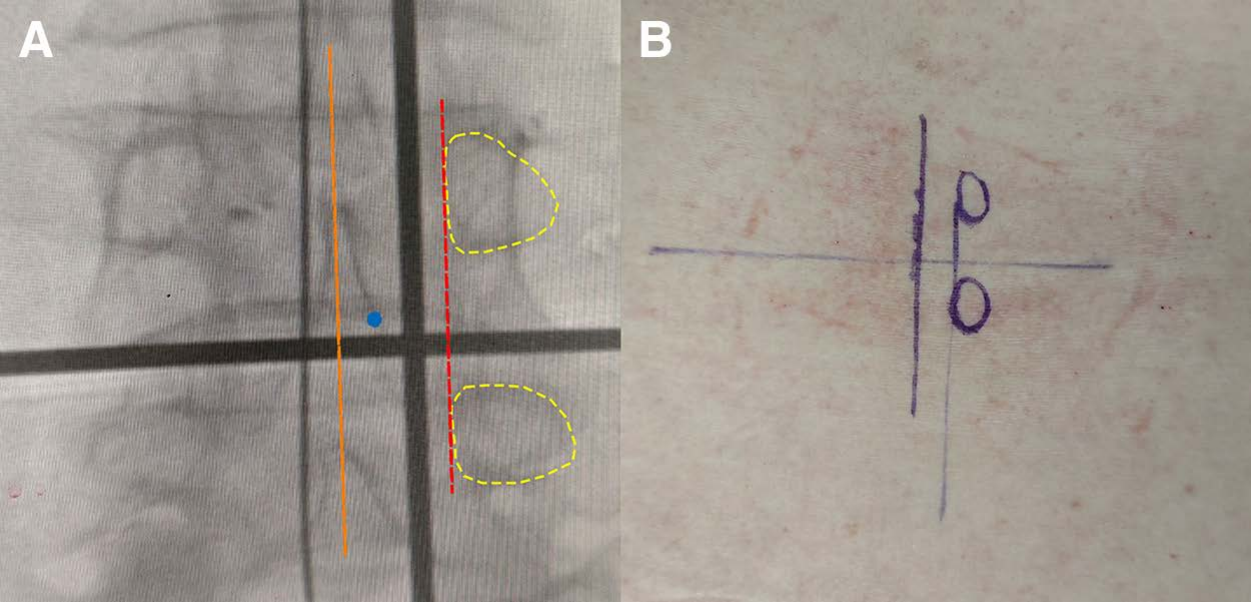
Preoperative X-ray localization and body surface marking. (A) Anteroposterior-X-ray fluoroscopy image of the surgical lumbar segment before operation, with Kirschner wires placed on the body surface for marking. The thin longitudinal Kirschner wire (orange line) indicates the spinous process line; the thick Kirschner wire (red line) indicates the line of the medial edge of the pedicle (all Kirschner wires are placed slightly to the left to avoid obscuring anatomical structures). The transverse Kirschner wire indicates the intervertebral space level; the blue dot indicates the initial point; the yellow line represents the pedicle contour. (B) Corresponding markings made on the body surface based on the preoperative fluoroscopy images.
